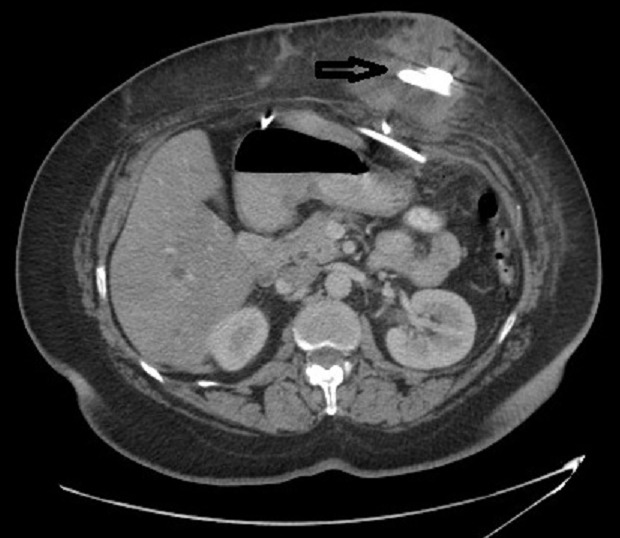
Abdomen CT scan showed fluid collection around the access-port (arrow)
